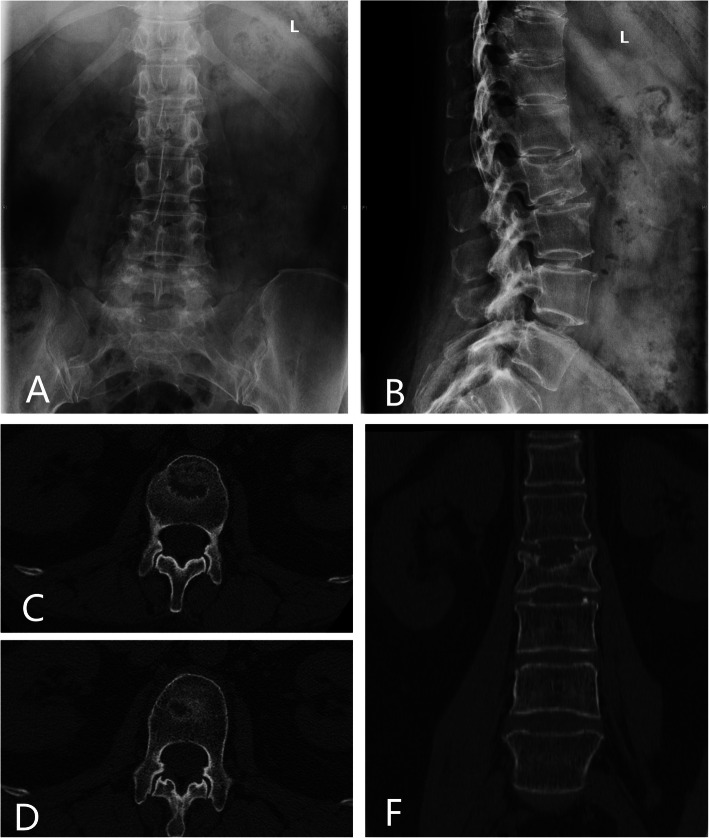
Anteroposterior (a) plain X-ray of lumbar spine showed no abnormalities. Lateral plain X-ray (b), axial (c, d), and coronal (f) CT images of the lumbar spine demonstrate a Schmorl’s node in the inferior endplate of L2
